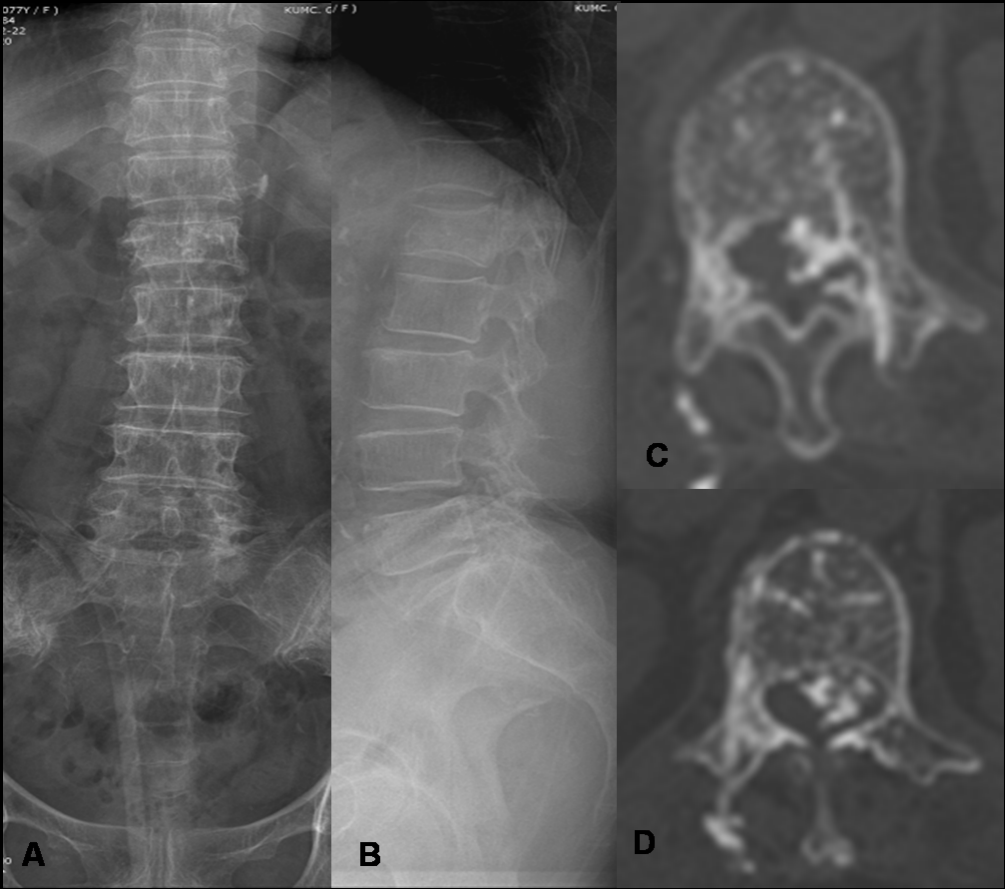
(A-B) shows post procedure radiogram of lumbar spine which did not exhibit extravasation if cement into the spinal canal at L1 level; figure 3 (C-D) shows CT scan of lumbar spine that exhibited epidural extension of cement from medial wall violation of L1 pedicle on left side and causing significant compression of cord.
